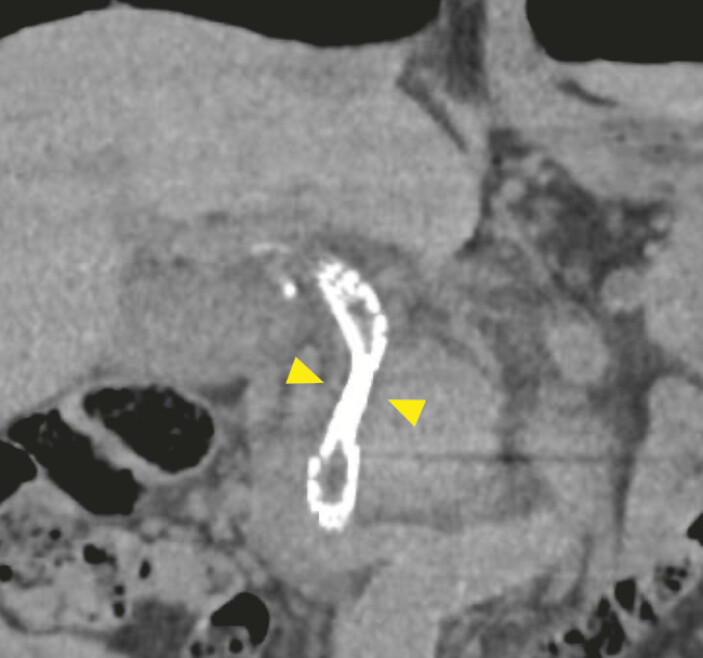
Abdominal computed tomography performed 16 days after endoscopic ultrasound-guided biliary drainage demonstrated the incomplete expansion of the antegrade metallic stent (yellow arrowheads).
